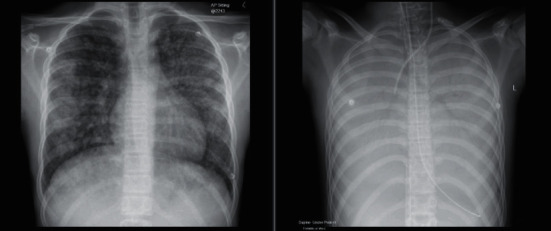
Early and late chest radiograph's demonstrating pulmonary haemorrhage.
